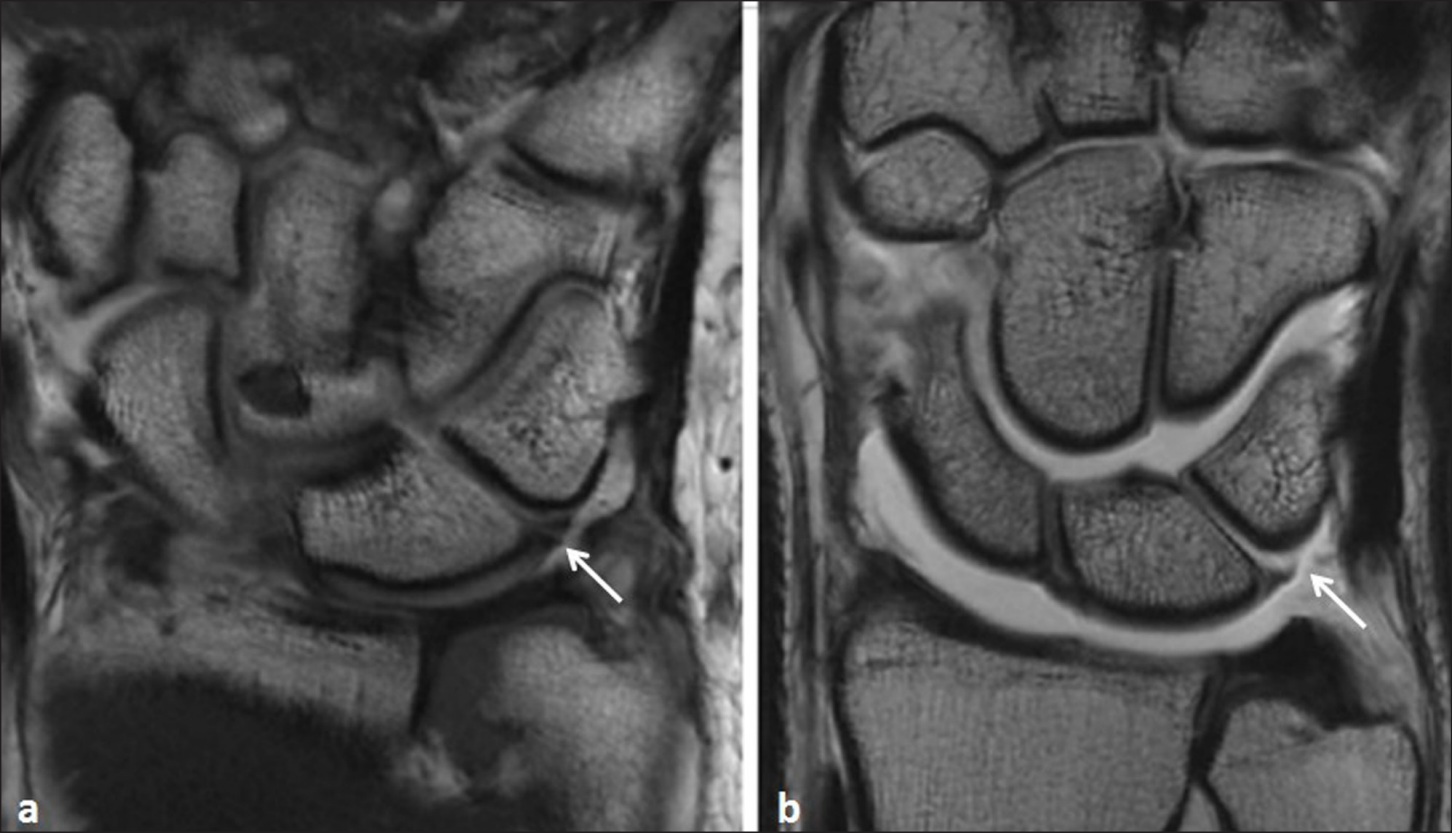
T1-weighted images in the coronal plane in two symptomatic patients with a lunotriquetral ligament tear. a. Without traction. The tear is visible as a thin interruption of the ligament (*arrow*). b. With traction. In this case, the tear is distended by contrast medium (*arrow*).
